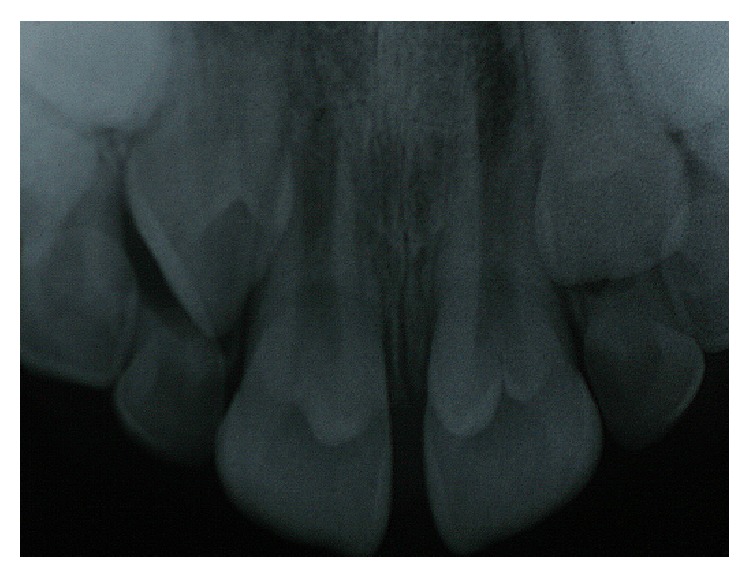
Radiographic appearance of the talon cusp.
